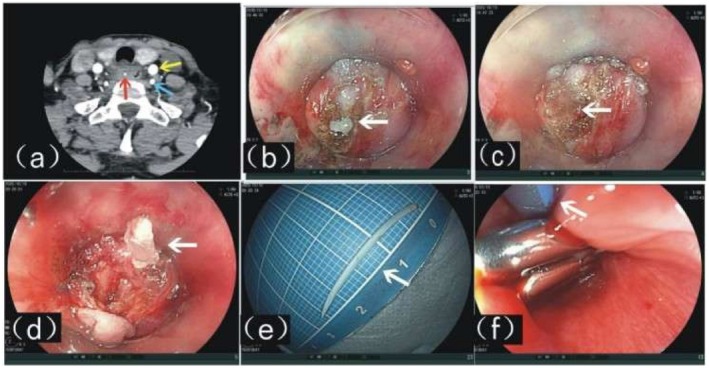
(a) Contrast‐enhanced CT demonstrating the foreign body (red arrow) and its relationship to adjacent vessels, including the common carotid artery (yellow arrow) and vertebral artery (blue arrow). (b) Endoscopic mucosal incision with drainage of purulent material (white arrow). (c) Identification of the embedded fish bone within the abscess cavity (white arrow). (d) Enlargement of the incision to expose the foreign body (white arrow). (e) Extracted fish bone measuring approximately 2.5 cm (white arrow). (f) Post‐procedural closure with clips and placement of a gastric tube (white arrow).
